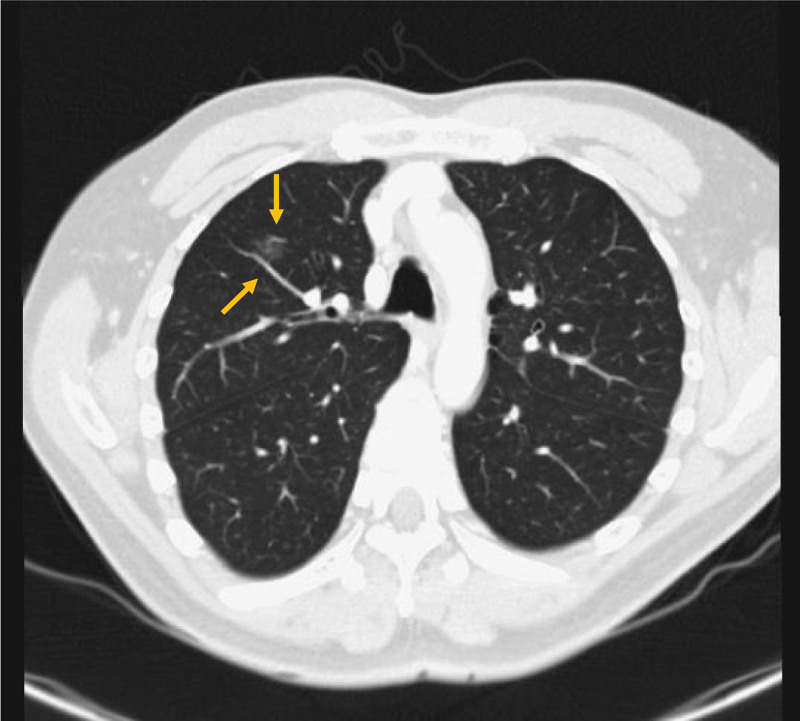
The patient previous chest computed tomography reveals that the ground grass opacity is located proximal to a vein that drains back to the pulmonary vein; the diameter of the vein is 0.25 cm.
